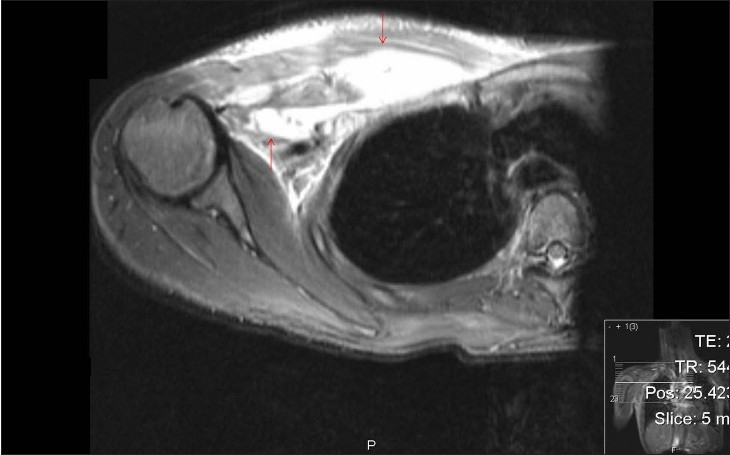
T2-weighted coronal magnetic resonance image of the right thorax. Note the abscess deep to the right pectoralis major muscle extending into the axilla (arrows)
